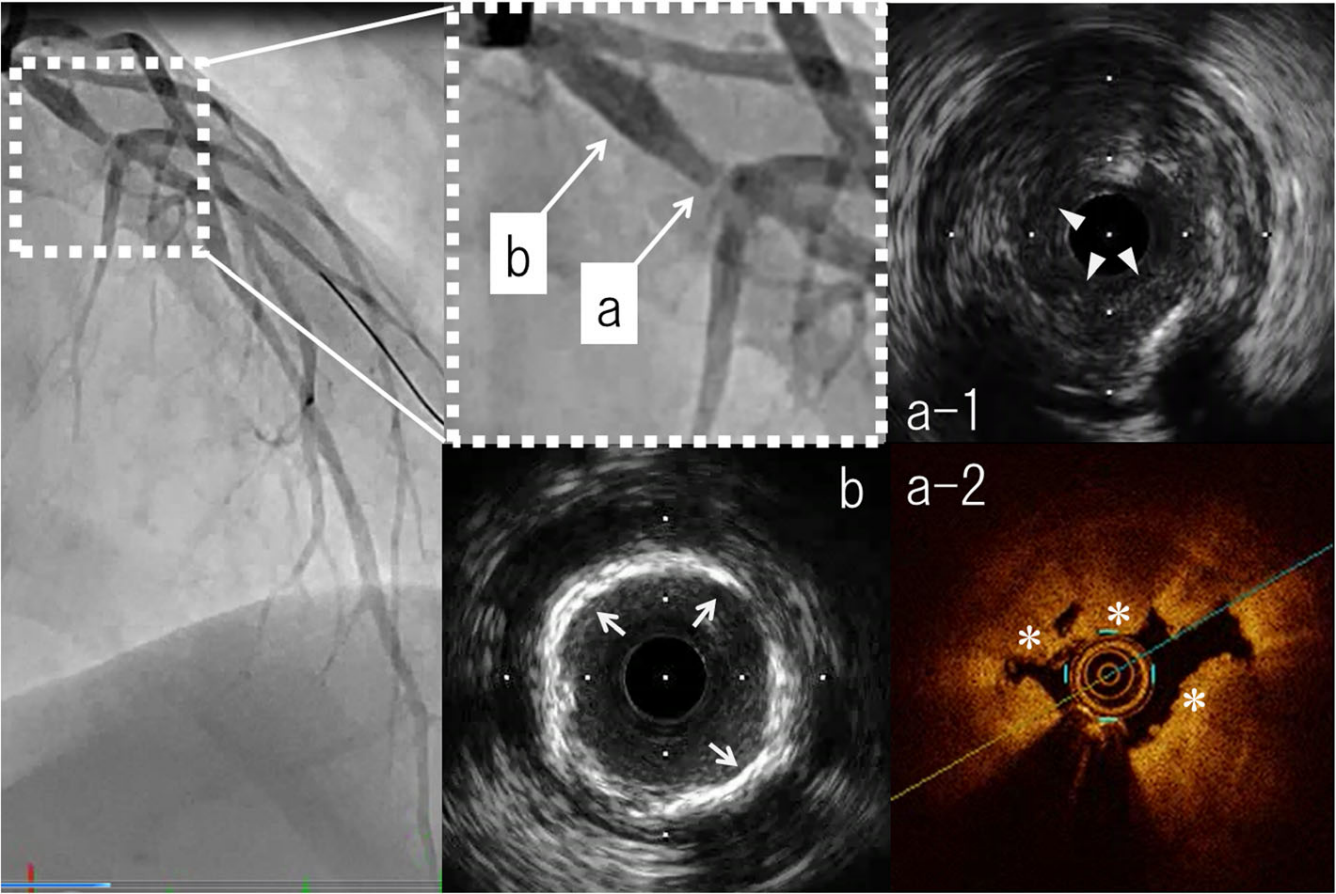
Intracoronary images using intravascular ultrasound (IVUS) and optical coherent tomography (OCT). a-1 At the occlusive site, intravascular ultrasound (IVUS) demonstrated a low-intensity structure with an irregular surface (arrow heads), suggesting the presence of a thrombus a-2 Optical coherent tomography (OCT) revealed that the thrombus showed strong signal attenuation and obscured underlying vascular structures (asterisk), suggesting the presence of a red thrombus, mostly consisting of red blood cells. b Proximal to the occlusive site, IVUS demonstrated a high intensity of the luminal surface and signal attenuation (arrows), indicating the presence of atherosclerotic plaque with calcification
